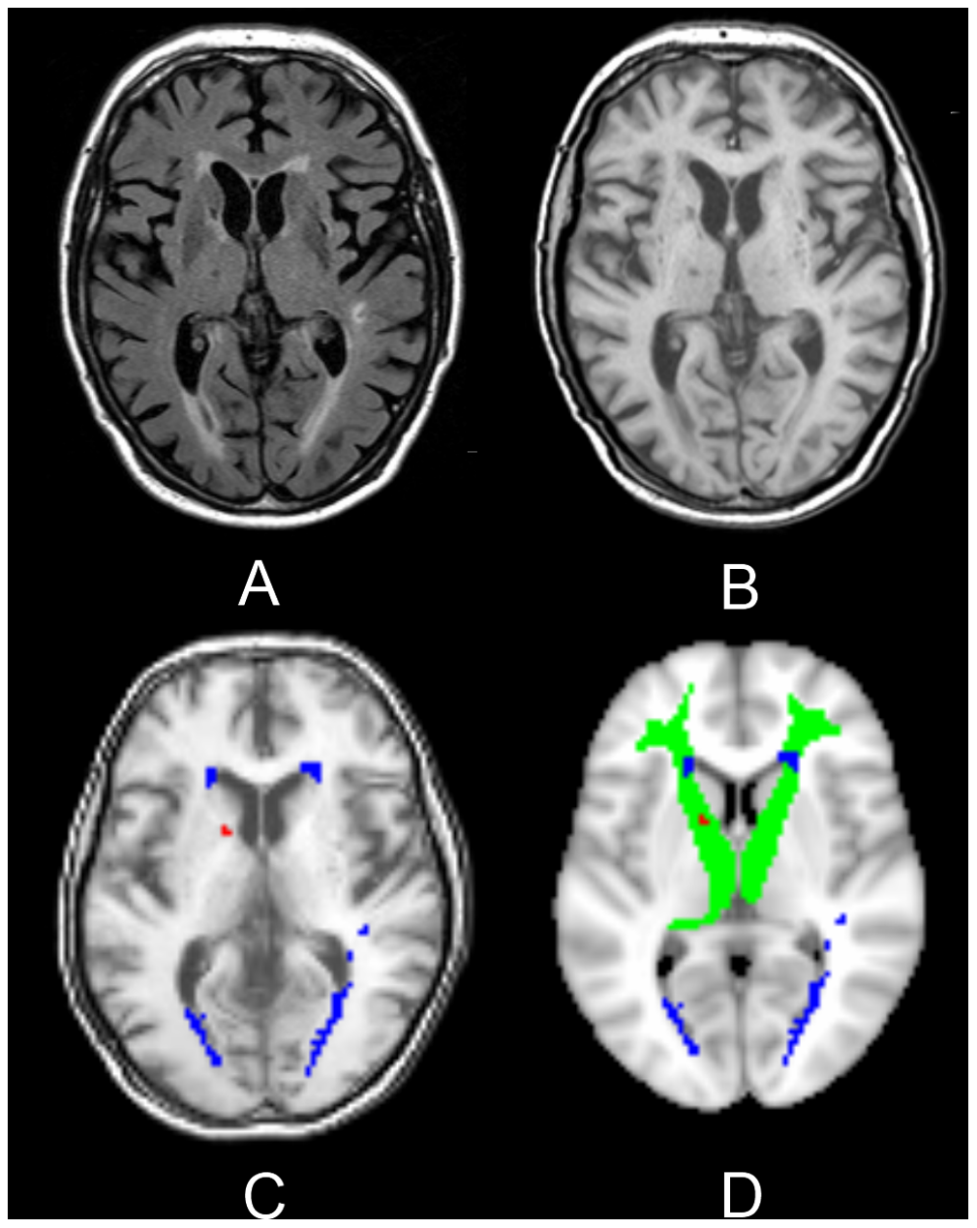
Segmentation of lesions within a specific white matter tract. The native T2 FLAIR (A) and T1 (B) sequences were used for segmentation of white matter lesions (WMLs) and lacunar lesions (LLs). The native T1 sequence was subsequently registered to MNI space followed by co-registration of the lesion maps using the same warp field. C: WMLs (blue) and LLs (red) projected on the registered T1 sequence. D: WMLs (blue) and LLs (red) projected on the MNI template. The anterior thalamic radiation is depicted in green. Regional lesion volumes within specific white matter tracts, in this case the anterior thalamic radiation, were associated with cognitive functioning using a linear regression model.
